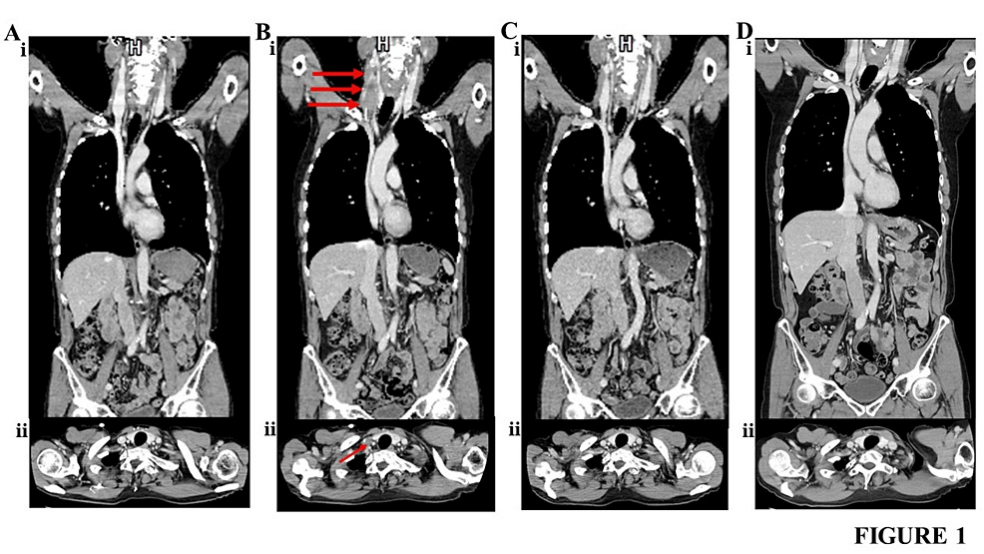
Contrast-enhanced CT findings in Case 1 (A) CT findings obtained on July 29, 2021. (i) Systemic findings and (ii) sixth cervical vertebrae findings. (B) CT findings obtained on August 20, 2021. (i) Systemic findings and (ii) sixth cervical vertebrae findings. (C) CT findings obtained on September 25, 2021. (i) Systemic findings and (ii) sixth cervical vertebrae findings. (D) CT findings obtained on January 4, 2024. (i) Systemic findings and (ii) sixth cervical vertebrae findings. Arrows indicate the thrombus in the right internal jugular vein at the CV catheter insertion site in the CV port. CT: computed tomography; CV: central venous
